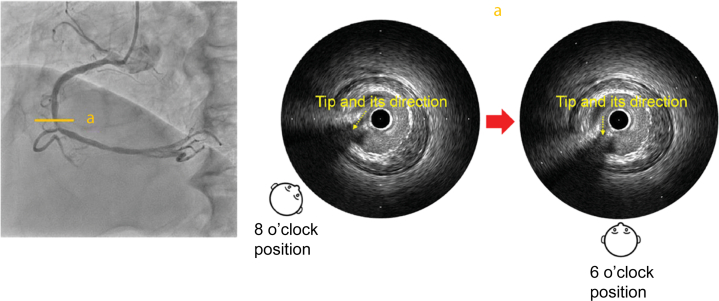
Unification of Viewpoints in Line With the Course of the Blood Vessel IVUS image from point a. Because the mid-RCA runs up to down, the IVUS image is rotated such that the operator's viewpoint is in the 6 o'clock direction with respect to the image. IVUS = intravascular ultrasound; RCA = right coronary artery.
